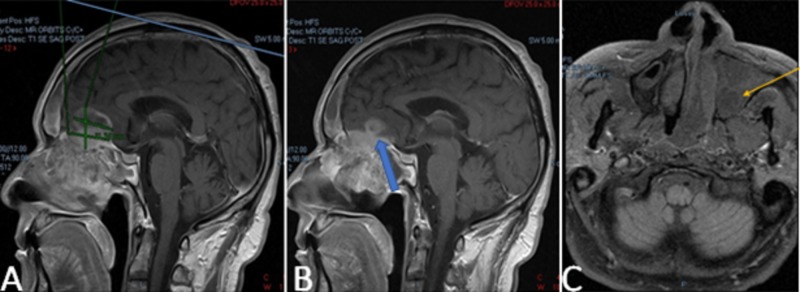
Magnetic resonance imaging (MRI) of the brain and orbits MRI of the brain and orbits demonstrates an aggressive, destructive, heterogeneously predominantly cystic sinonasal malignancy. A. Sagittal MRI revealing ethmoid sinus involvement spreading to the brain. B. Interval intracranial extension through destruction of cribriform plate (blue arrow). C. Midline irregular mass adherent to nasal septum, with septum deviation and surrounding edema (yellow arrow).
